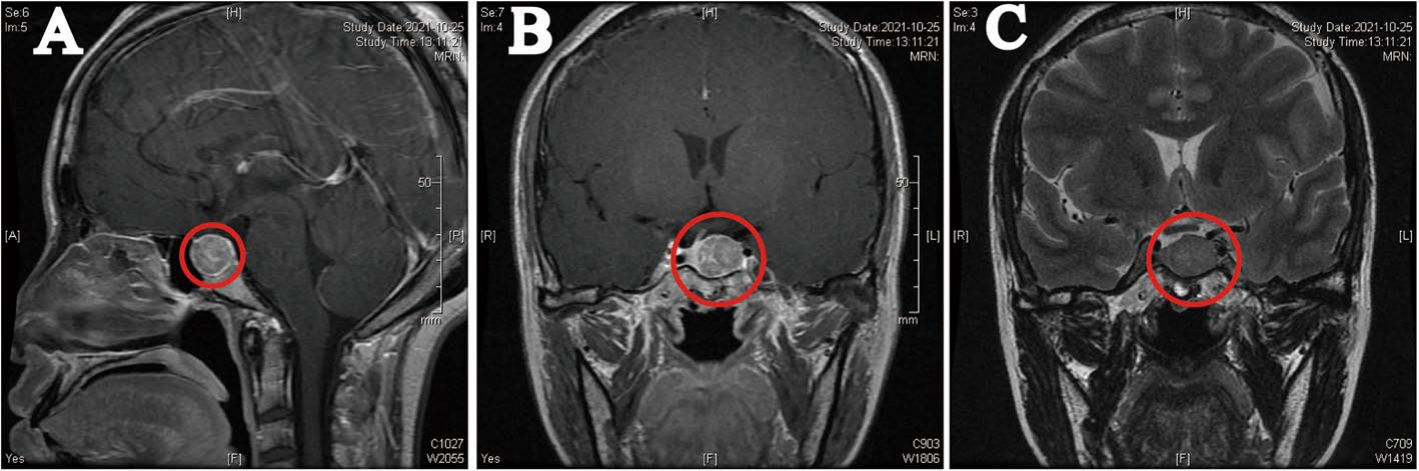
Brain magnetic resonance imaging. There was a pituitary macroadenoma (18 × 16 × 16 mm) with a quasi-circular equal signal shadow in the sellar region. A. gadolinium-enhanced sagittal T1WI, B. gadolinium-enhanced coronal T1WI, C. coronal T2WI
